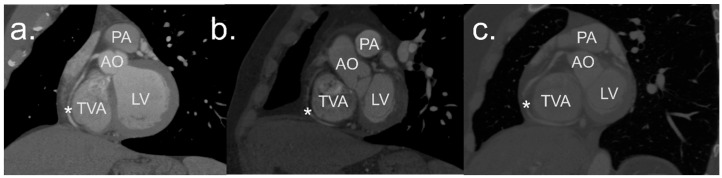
Multiplanar reconstruction of computed tomography angiography visualizing the tricuspid valve annulus area. (a) View of the tricuspid valve annulus region from RAO 60°, caudal 5°; (b) RAO 40°, caudal 0°; (c) RAO 45°, caudal 10°. The right coronary artery is marked with *. AO—aorta, PA—pulmonary trunk, TVA—tricuspid valve annulus, LV—left ventricle.
